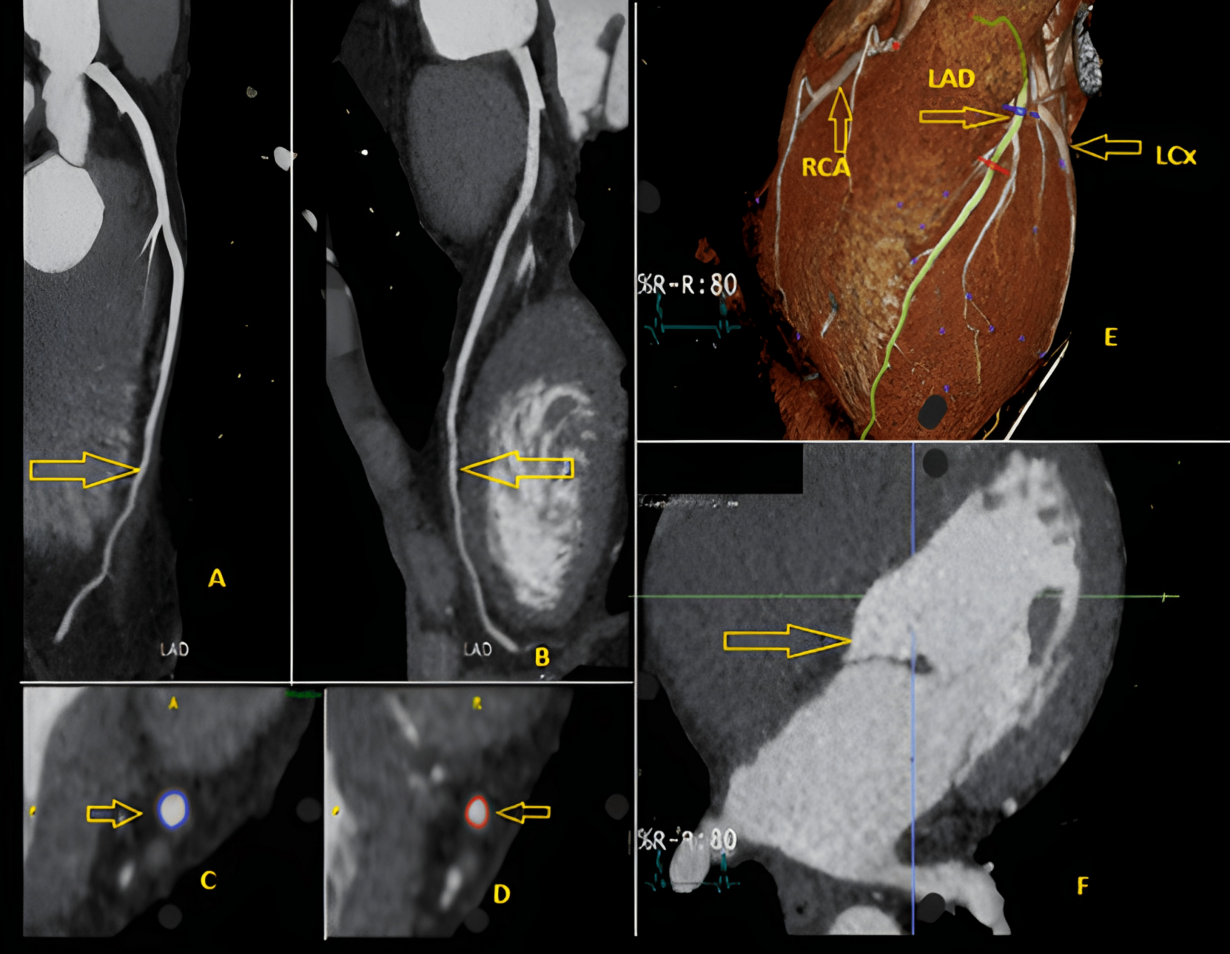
CT coronary angiogram (performed four years prior) showing absence of coronary calcification and a small non-obstructive plaque in the distal left anterior descending artery. (A–D) Curved multiplanar reformations of the left anterior descending (LAD) coronary artery demonstrating a patent vessel with mild, non-obstructive distal atherosclerotic plaque (yellow arrows). No flow-limiting stenosis or high-risk plaque features are identified. (E) Three-dimensional volume-rendered reconstruction showing the coronary tree, including the LAD, right coronary artery (RCA), and left circumflex artery (LCx), all without significant abnormalities. (F) Short-axis view of the left ventricle demonstrating normal cavity size and myocardial wall thickness.
